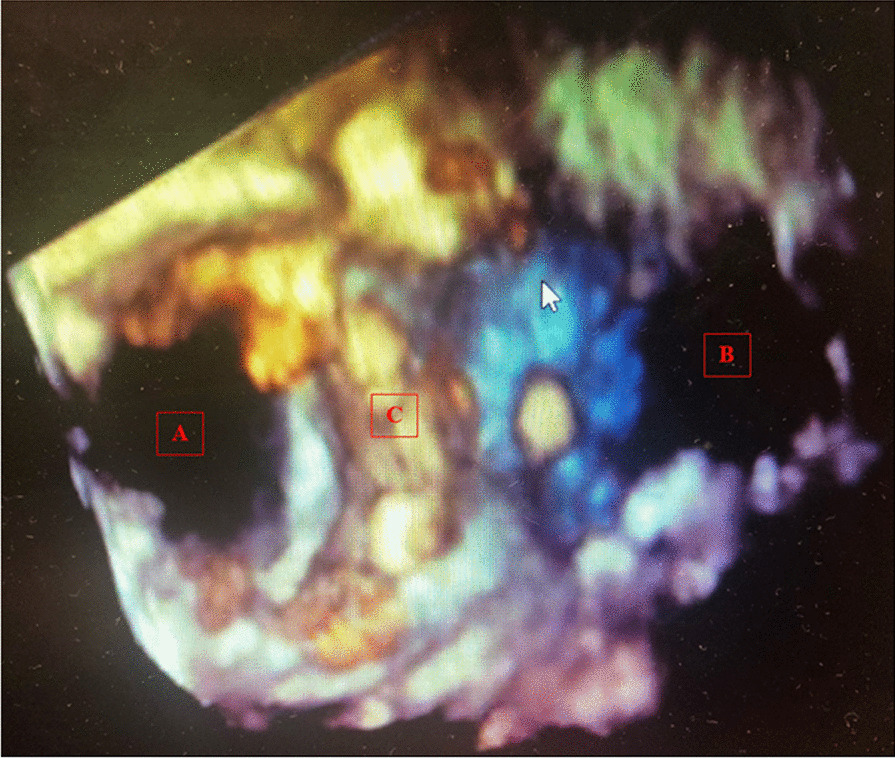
3D transeophageal echocardiogram image demonstrating both Sapien 3 and Intuity elite orifice from within the left ventricle with no impingement, (A) and (B) respectively. (C) Demonstrates the frame of the Sapien 3 prosthesis
